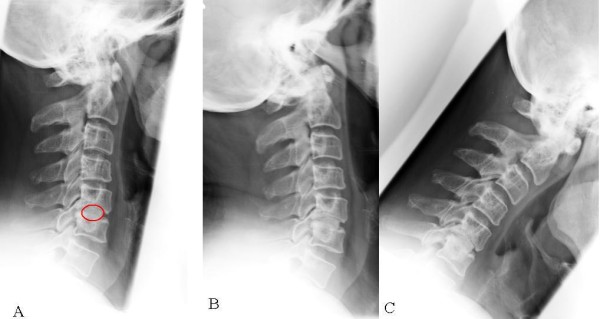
Exemplary plain (A), extension (B) and flexion (C) radiographs of a 57 year old patient with pseudarthrosis of the operated level and without signs of ASD 7 years after surgery. The operated pseudarthrotic level C5/6 is indexed, and the spheric form of the graft material has the aim to prevent migration (A).
